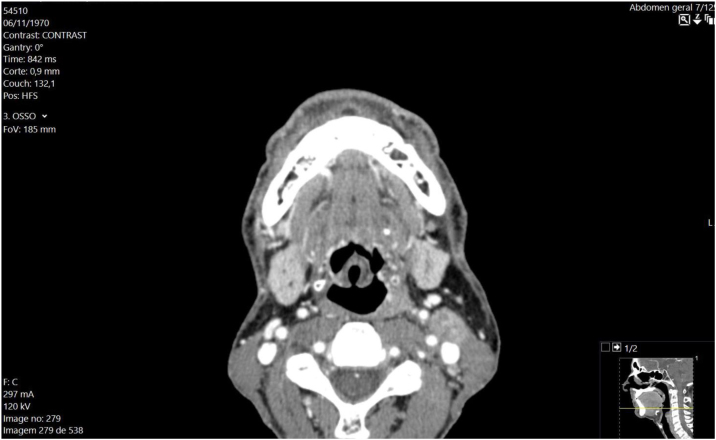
CT-Scan of head and neck showing omega epiglottis.
